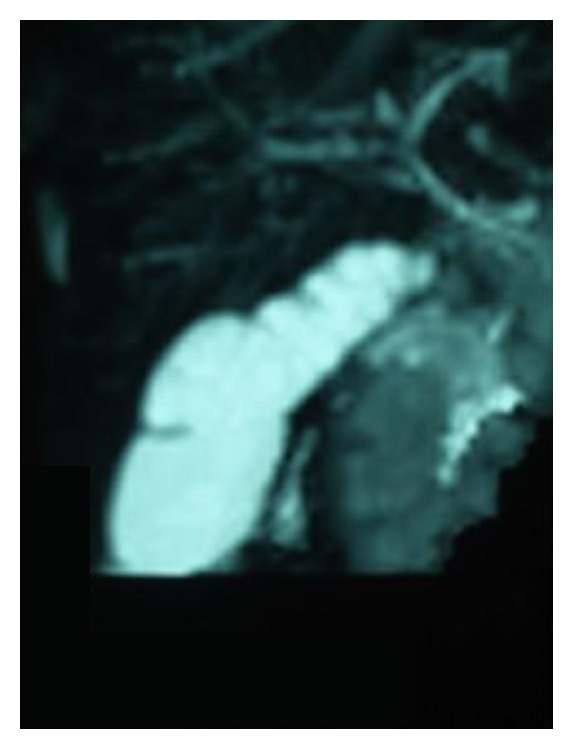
Magnetic Resonance cholangiopancreaticography (MRCP) image confirming ultrasound findings of multiple septae within the gallbladder.
